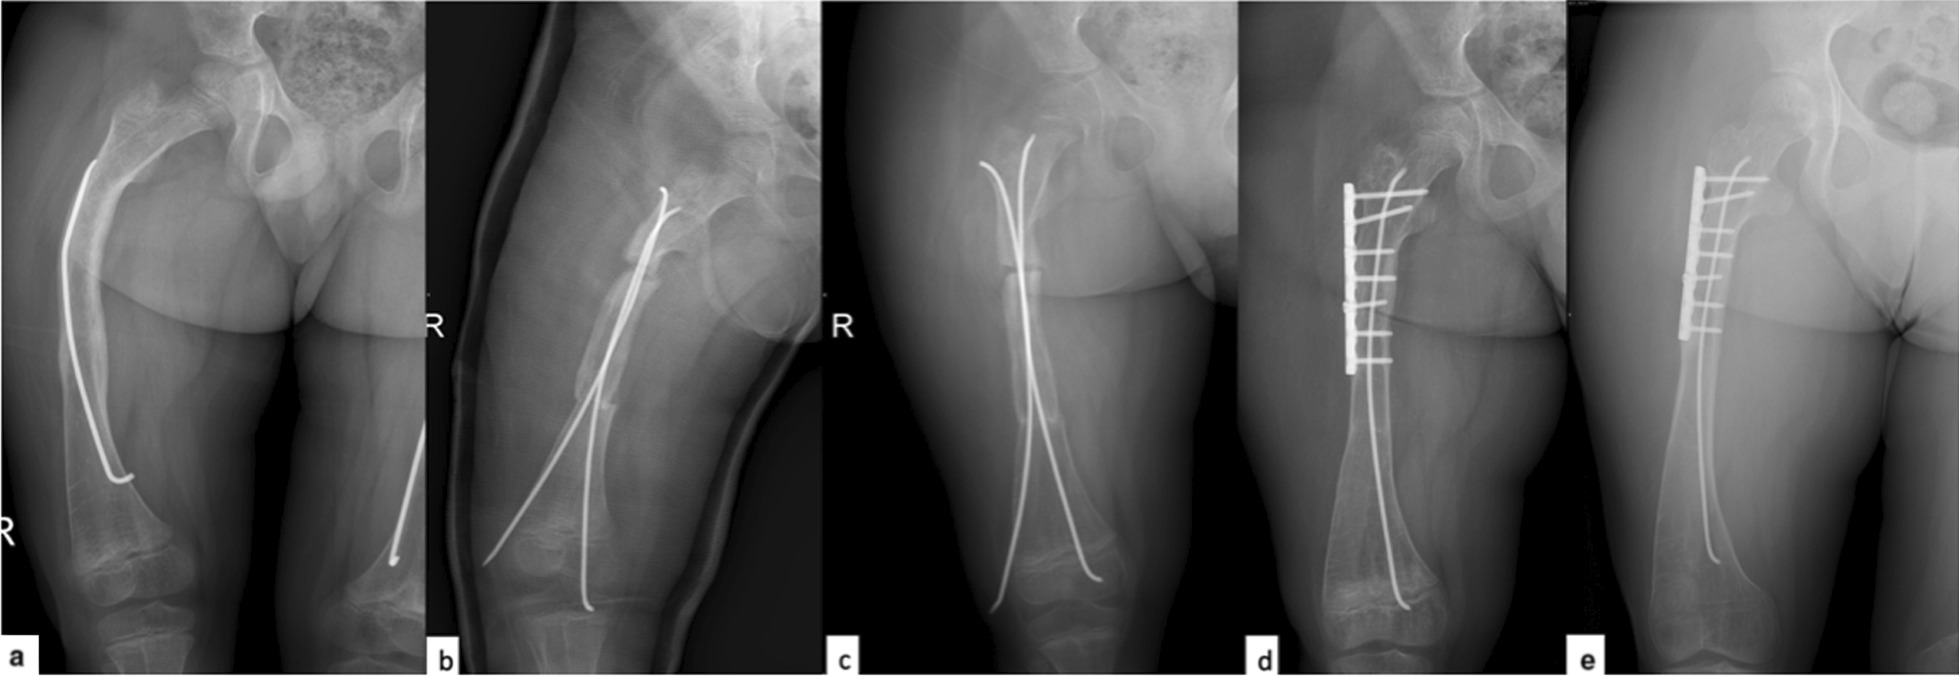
Patient with intramedullary fixation only a preoperative radiograph, b postoperative first-day radiograph, c postoperative sixth-month radiograph, union in the distal osteotomy of the patient but nonunion was seen in the proximal osteotomy, d second-year follow-up radiograph after revision surgery and plate fixation. Total union was seen and deformity recurrence was not observed, e third-year follow-up radiograph after revision surgery and plate fixation. Deformity recurrence was not observed
